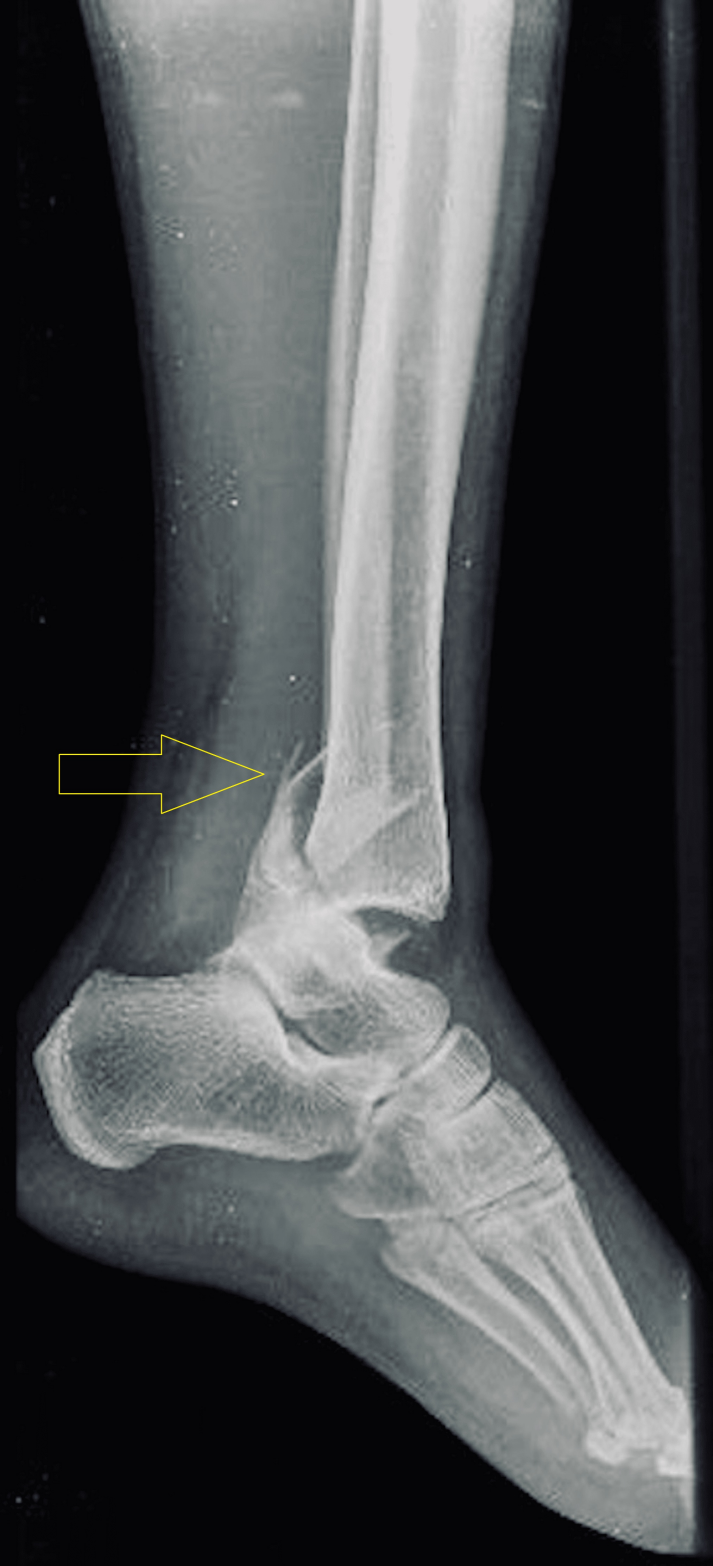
Pre-operative X-ray (lateral view) of the left ankle.
